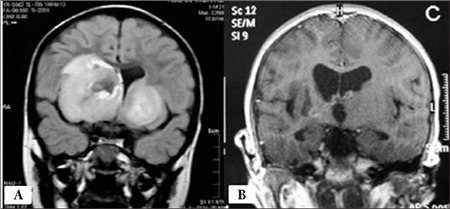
A) MRI of the brain showing 2 huge masses in the periventricular area. Mild brain edema was seen around the lesion. B) MRI showed complete resolution of the masses after treatment.
